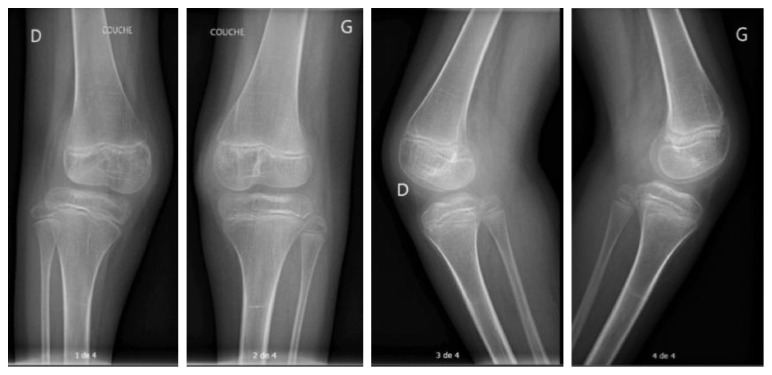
Patient 1: knee X-rays at follow-up (age 15).
